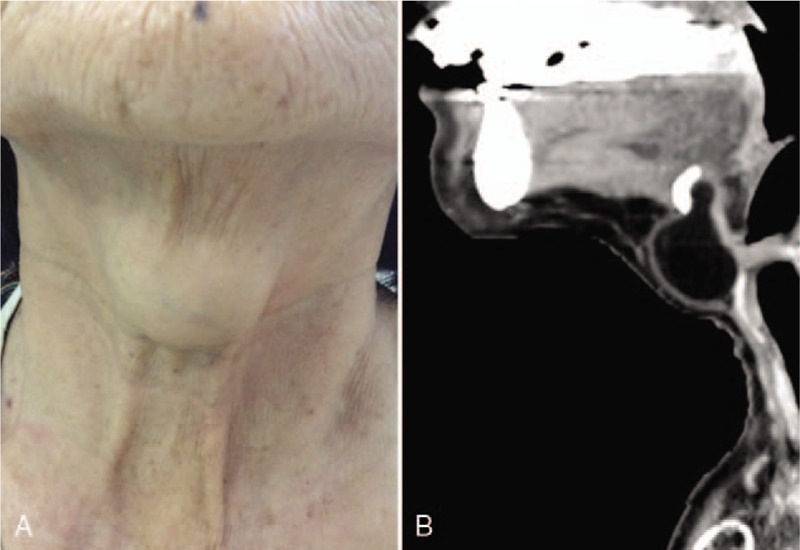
A 63-year-old female patient with thyroglossal duct lipoma. (A) Photograph of the patient presenting a midline neck mass (grossly 3.5 × 3.0 cm^2^) located between the thyroid cartilage and hyoid bone. (B) Sagittal view of the computed tomography image demonstrating a 3.5 × 3.0-cm^2^ well-circumscribed homogeneous ovoid mass with low attenuation with a ductal remnant to the tongue base.
